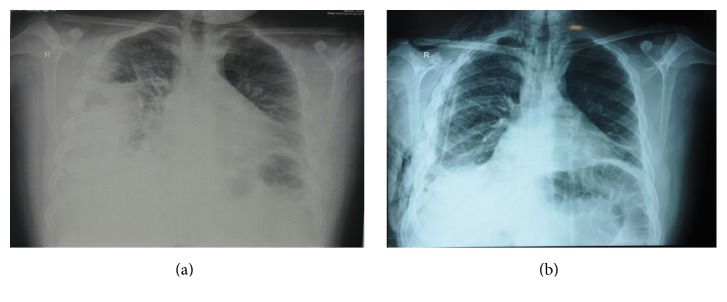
(a) Preoperative chest X-ray shows right sided obliteration of costophrenic angle and displaced right lung. (b) Postoperative resolution of the empyema (note chest tube).
